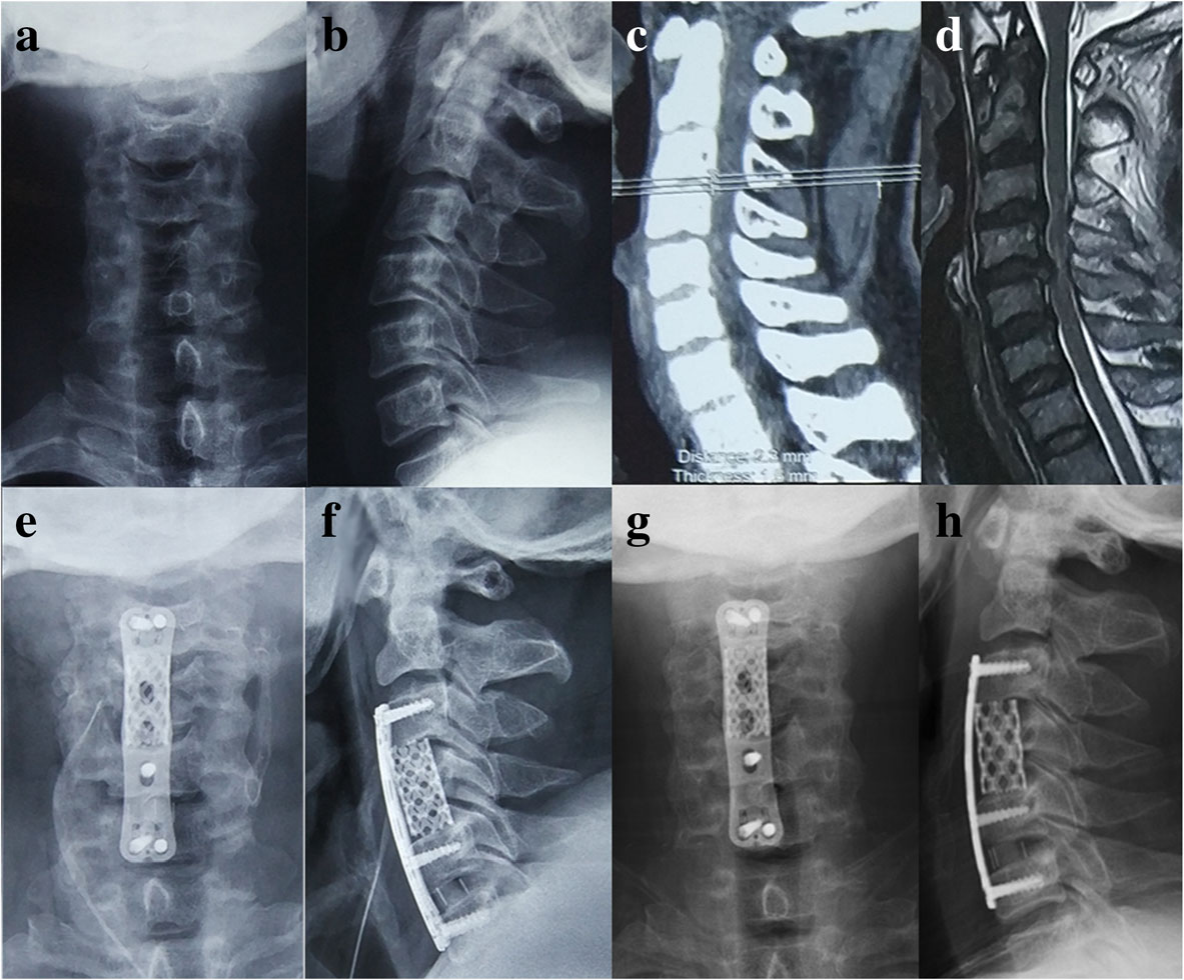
A 52-year-old male developed numbness and weakness in his four extremities for 2 years, together with unbalance gait for 2 months. Preoperative radiographs showed that the sagittal alignment of the cervical spine was physiologic lordosis (a, b), and the magnetic resonance imaging scans showed that the spinal cord compressed at C3/4, C4/5, C5/6 (c, d). He was performed with ACHDF including 1-level corpectomy plus 1-level discectomy without surgery-related complications. After operation, his JOA scores improved from 9.7 preoperation to 13.6 postoperation. Postoperative lateral and flexion-extension cervical radiographs showed that the cervical kyphosis was corrected (e, f) and the graft was fused at 1-year follow-up (g, h)
